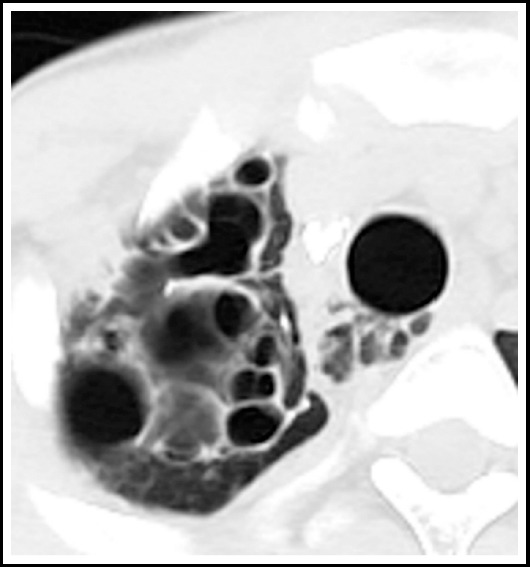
CT scan chest section in lung window setting shows loculated pneumomediastinum secondary to chronic cough. Saccular bronchiectatic changes are seen in the right upper lobe.
